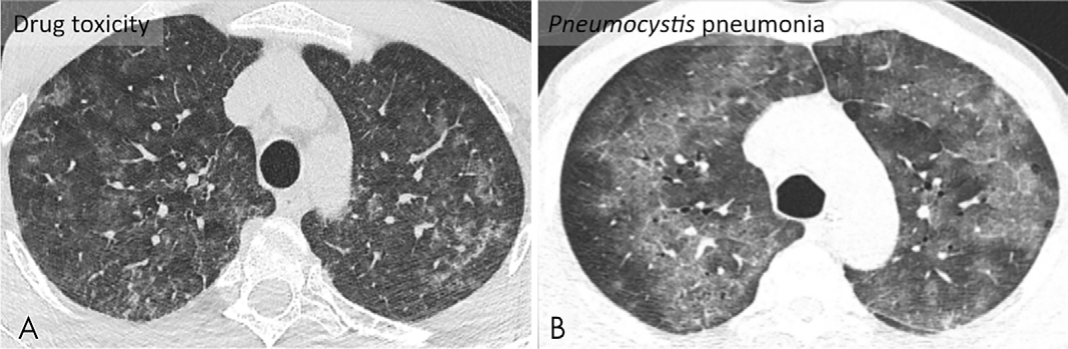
Indeterminate CT imaging features for COVID-19. Widespread GGO with nonrounded morphology and no specific distribution in unenhanced axial images from two different patients secondary to acute lung injury from presumed drug toxicity (*A*) and *Pneumocystis* pneumonia (*B*). Routine screening CT for diagnosis or exclusion of COVID-19 is currently not recommended by most professional organizations or the U.S. Centers for Disease Control and Prevention.
